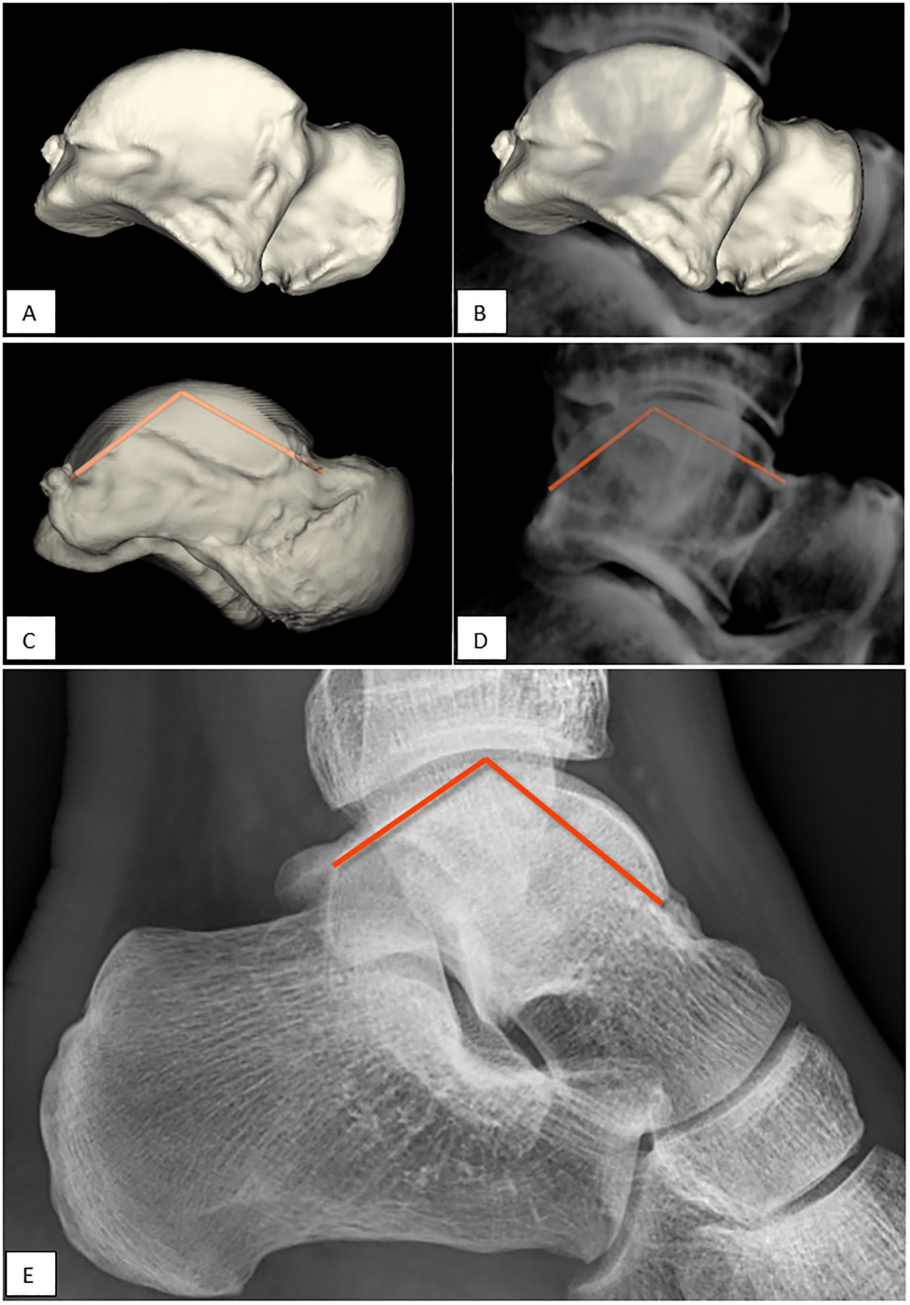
Validation of the TCA. (A) 3D polygon based on computed tomographic segmentation. (B) 3D polygon included in the DRR. (C) TCA measured between 2 lines in 3D space. (D) TCA measured on the DRR in 2D plane. (E) TCA measured on a standard lateral radiograph. DRR, digitally reconstructed radiograph; TCA, talar convexity angle.
